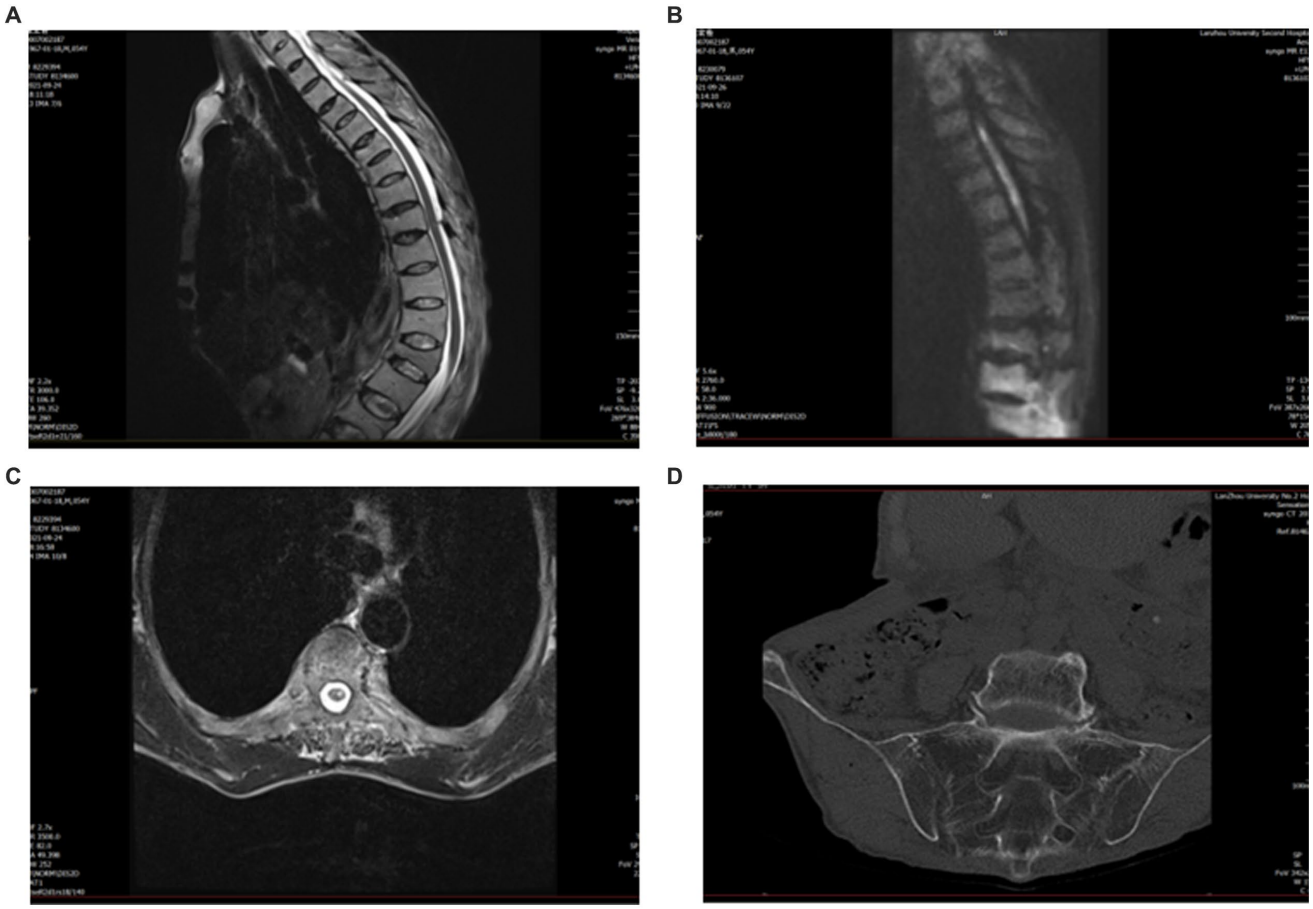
Pre-treatment magnetic resonance imaging (MRI). (A) Sagittal T2 cervical spine shows high signal T2–T5. (B) Diffusion-weighted imaging (DWI) shows restricted diffusion within the gray matter. (C) Axial T2 suggests a high signal centrally in the spinal cord. (D) CT of the sacroiliac joint shows bilateral sacroiliitis and calcification of the anterior and posterior longitudinal ligaments.
